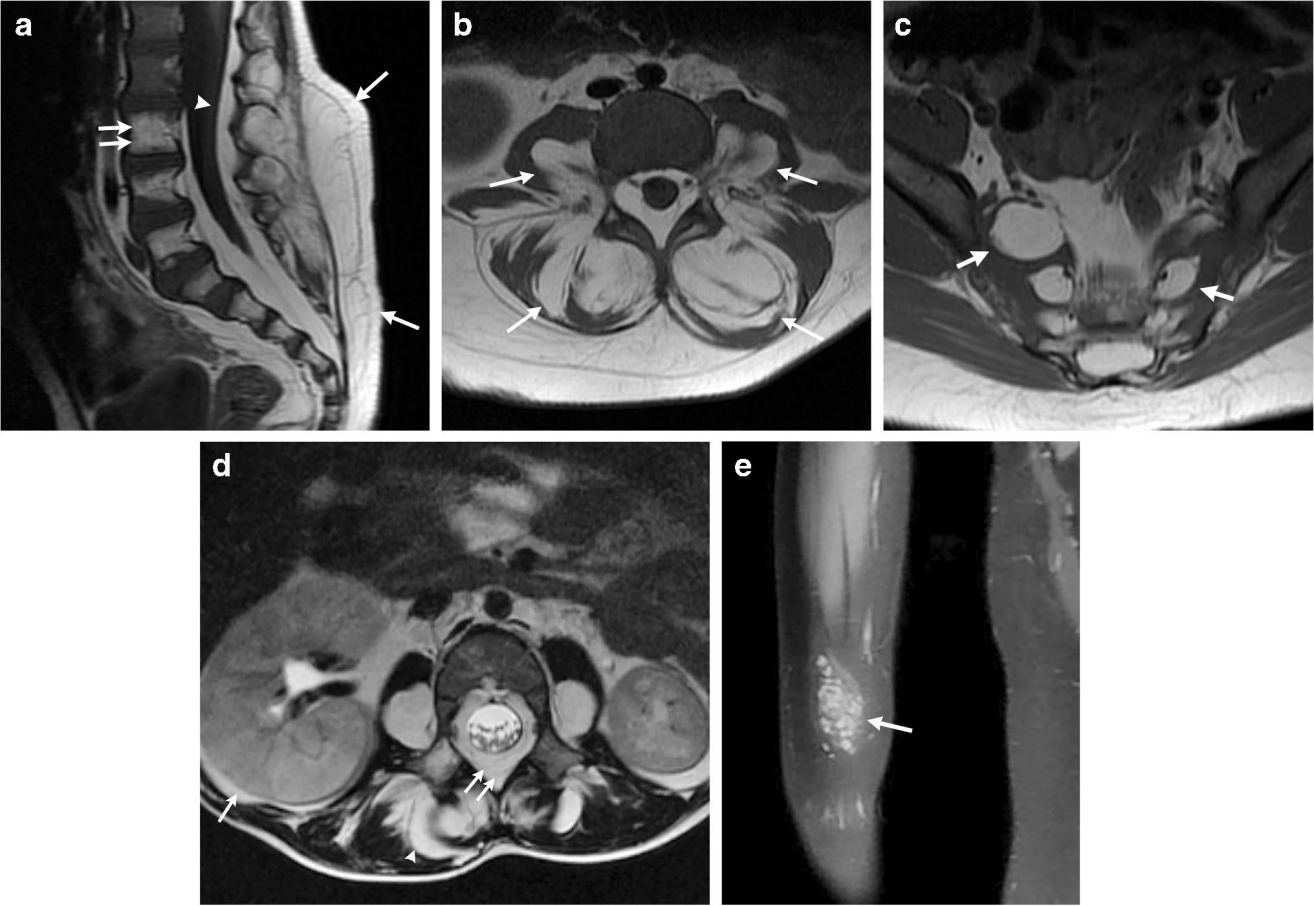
Bannayan-Riley-Ruvalcaba syndrome in a 3-year-old boy with a genetically confirmed *PTEN* mutation with multiple anomalies. a-c Sagittal and axial T1-weighted images show excessive fat throughout the lumbosacral spine. In (a), there is cutaneous and subcutaneous dorsal lipomatous overgrowth (*arrows*), extensive dorsal and ventral epidural fat (*arrowhead*) and abnormal fat within the vertebral body bone marrow (*double arrows*). In (b, c), extensive fatty replacement is observed within the psoas and paraspinal muscles (*arrows* in b) and along the sacral neural foramina (*arrows* in c). d An axial T2-weighted image shows right-side nephromegaly (*arrow*) as well as the excess fat in the paraspinal tissues (*arrowhead*) and epidural fat (*double arrows*). e A coronal contrast-enhanced T1-weighted image shows a solid enhancing mass in the ventral forearm consistent with a venous malformation
